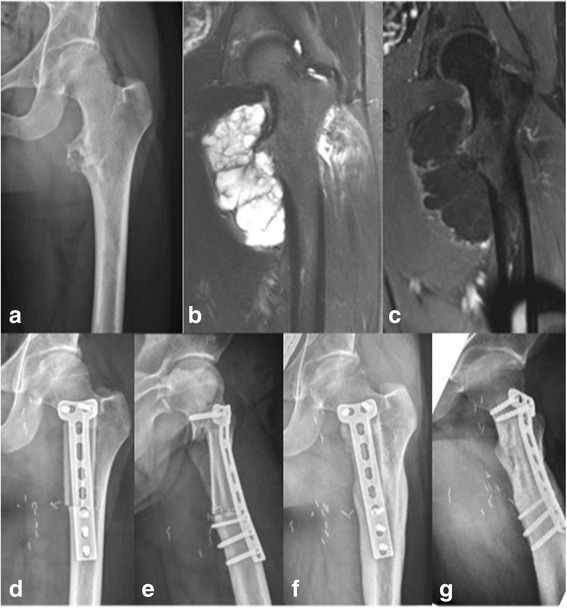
Preoperative imaging of a 28-year-old male patient with periosteal chondrosarcoma (G2) of the left proximal femur: conventional X-rays a STIR MRI sequence b e-Thrive MRI sequence with contrast agent c. Postoperative conventional antero-posterior d and latero-lateral e X-rays following wide resection and hemicortical reconstruction with two non-vascularised fibula struts. Complete integration of both struts and remodelling of the resected segment on conventional X-rays was observed 10 months after surgery f, g
